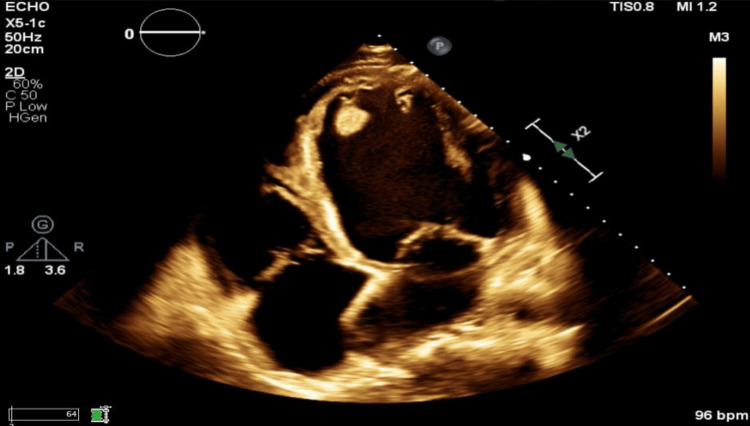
Transthoracic echocardiography, four-chamber view Transthoracic echocardiography in a four-chamber view showing a severely dilated left ventricle. A prominent apical thrombus is noted.
